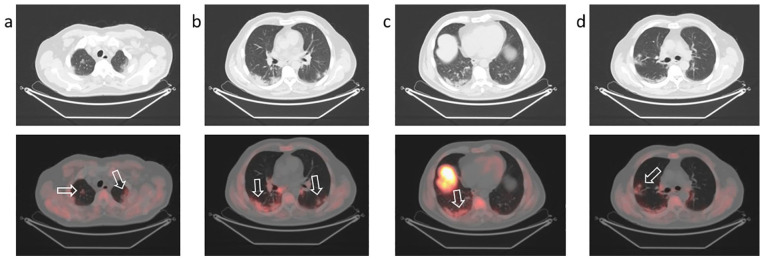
Positron emission tomography/computed tomography with ^18^F-choline
